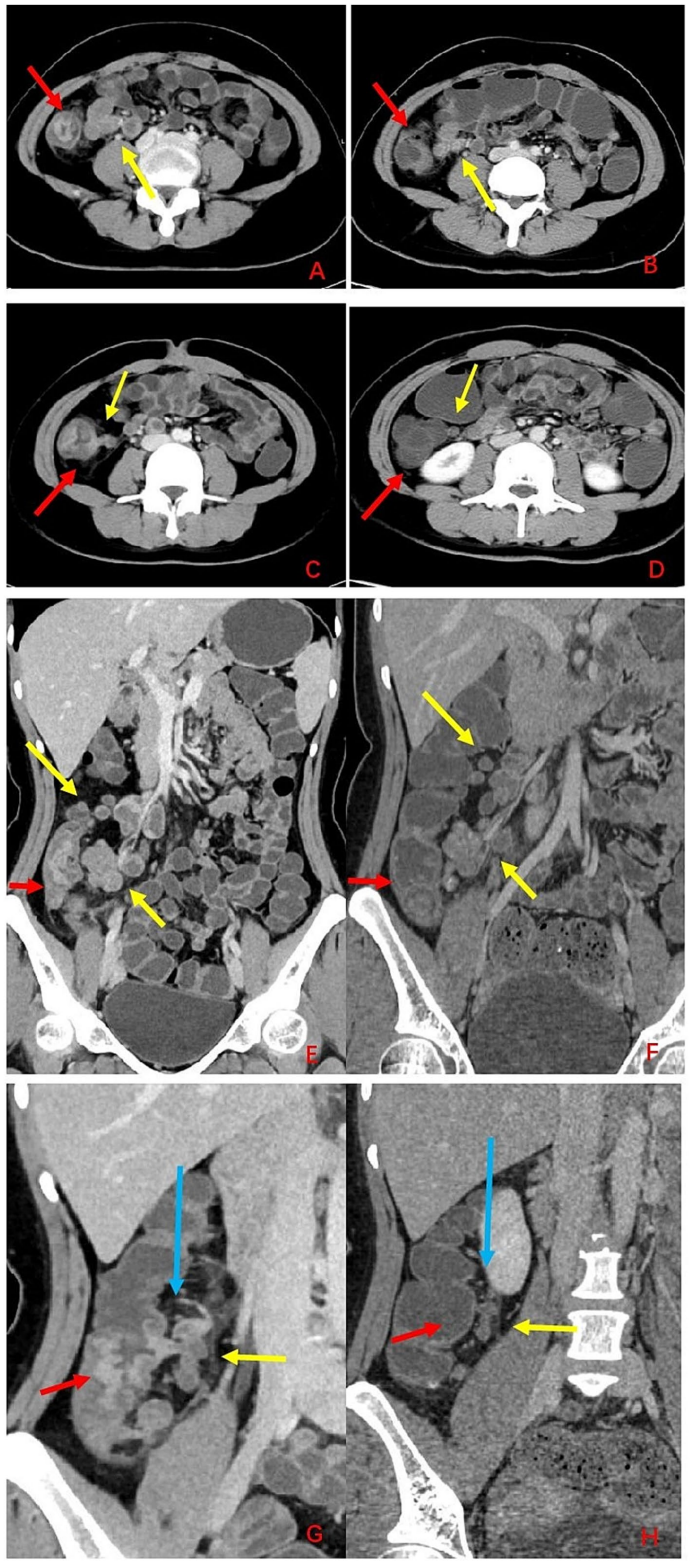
CT imaging findings before and after treatment. (A,C,E,G) Pre-treatment CT images. (B,D,F,H) Post-treatment CT images. Red arrows indicate lesions; yellow arrows indicate lymph nodes; blue arrows indicate sinus tract.
